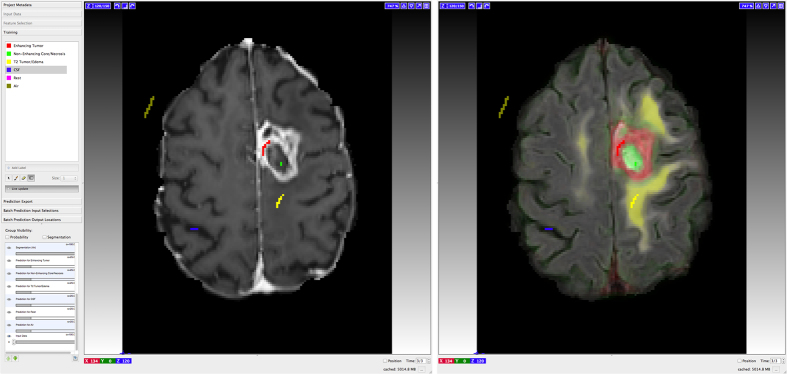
Graphical user interface of the presented software tool. The left side displays a contrast-enhanced T1w image of a GB patient; the corresponding FLAIR image with an overlay of predicted class probabilities is shown on the right. Annotations (Scribbles) are displayed in different colors: contrast enhancement (red), non-enhancing tumor (green), edema (yellow), CSF (blue) and air (dark yellow). For a demonstration please see Video 1.
